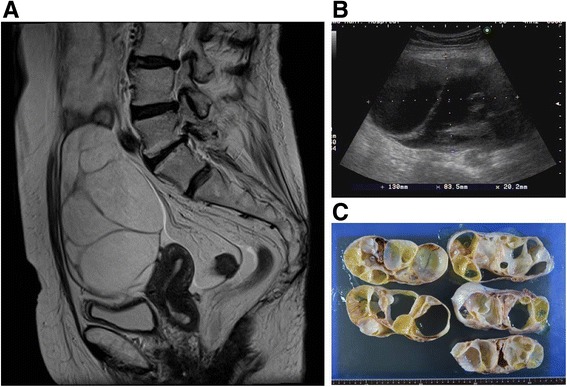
a Sagittal T2-weighted MRI shows a multilocular cystic mass, which is clearly delineated in the pelvis (maximum diameter approximately 20 cm). The uterus measures 80 mm in height, 55 mm in body length, and 6 mm in cavity thickness. b An ultrasound demonstrates the pelvic mass (diameter approximately 13 cm) containing a multilocular cyst without mural nodules. c Gross appearance, the tumor measures 19.0 cm x 14.0 cm x 8.5 cm. The inner surface of the cyst is mostly smooth, with areas of irregular thickening of the cyst wall, including yellowish mucoid substance
